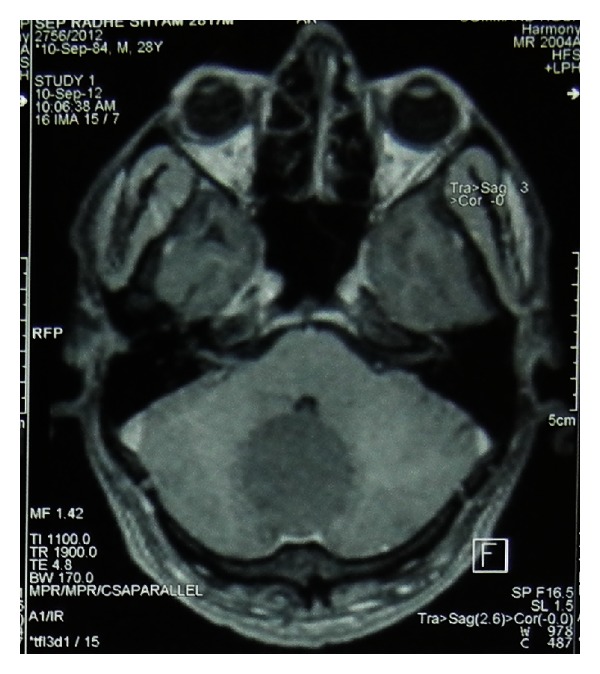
MRI (axial view) Showing mass in posterior fossa.
